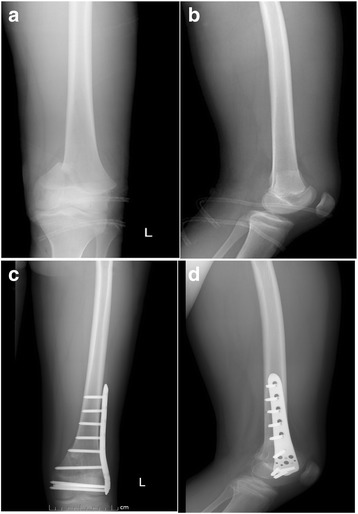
Preoperative and immediate postoperative images of X-rays. a, b Preoperative X-rays anteroposterior and lateral views of knee joint showing distal femur fracture. c, d Immediate postoperative anteroposterior and lateral X-rays showing fracture consolidation
